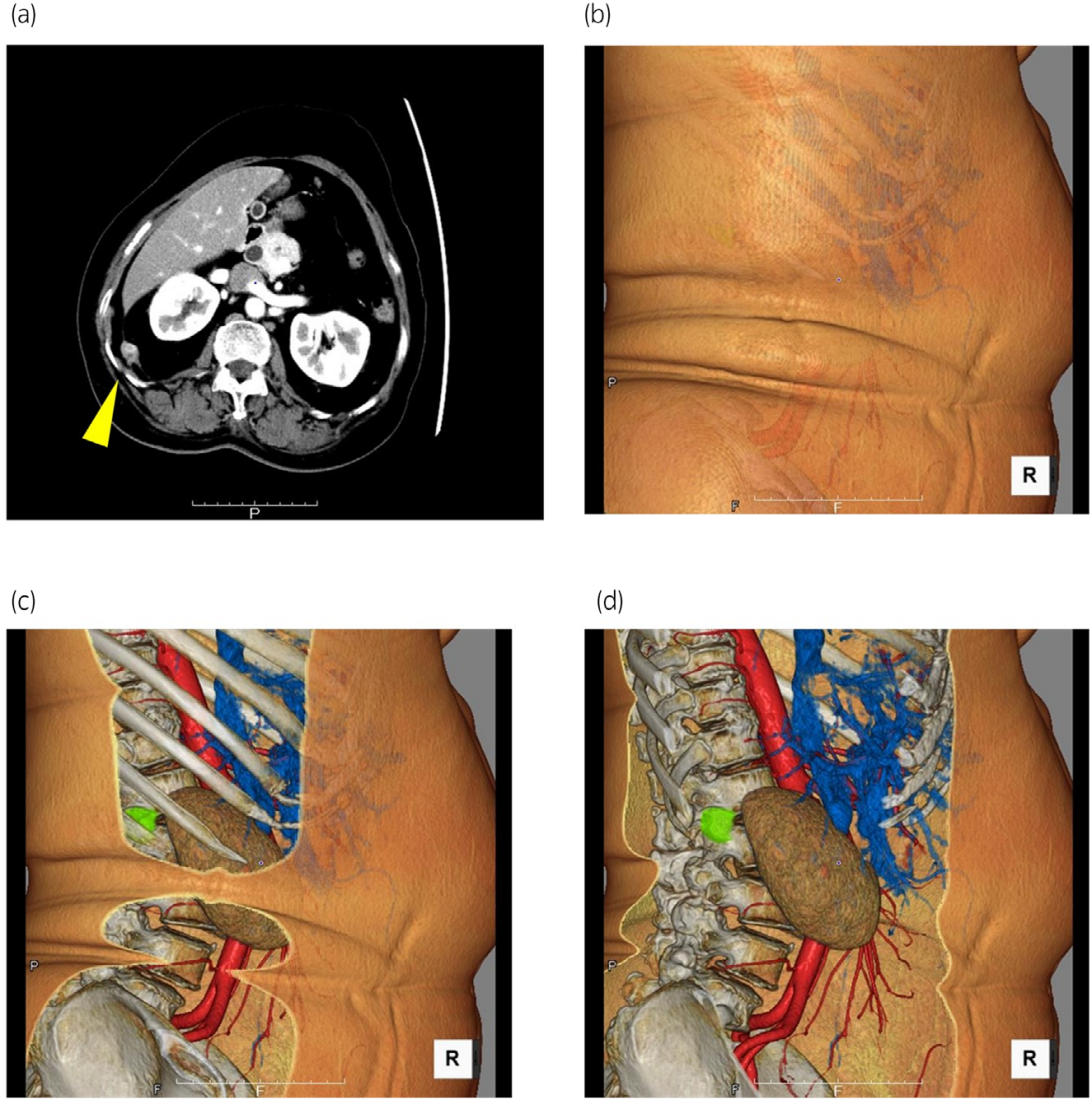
(a) A contrast‐enhanced CT scan shows retroperitoneal metastasis in the lateroconal fascia close to the right diaphragm, partly infiltrating the peritoneum. The tumor measured 2 cm in size and showed contrast enhancement. (b–d) STL data (3D data) were produced using the workstation. (b–d) 3D data from the skin to inside the body (the green sphere indicates the tumor).
